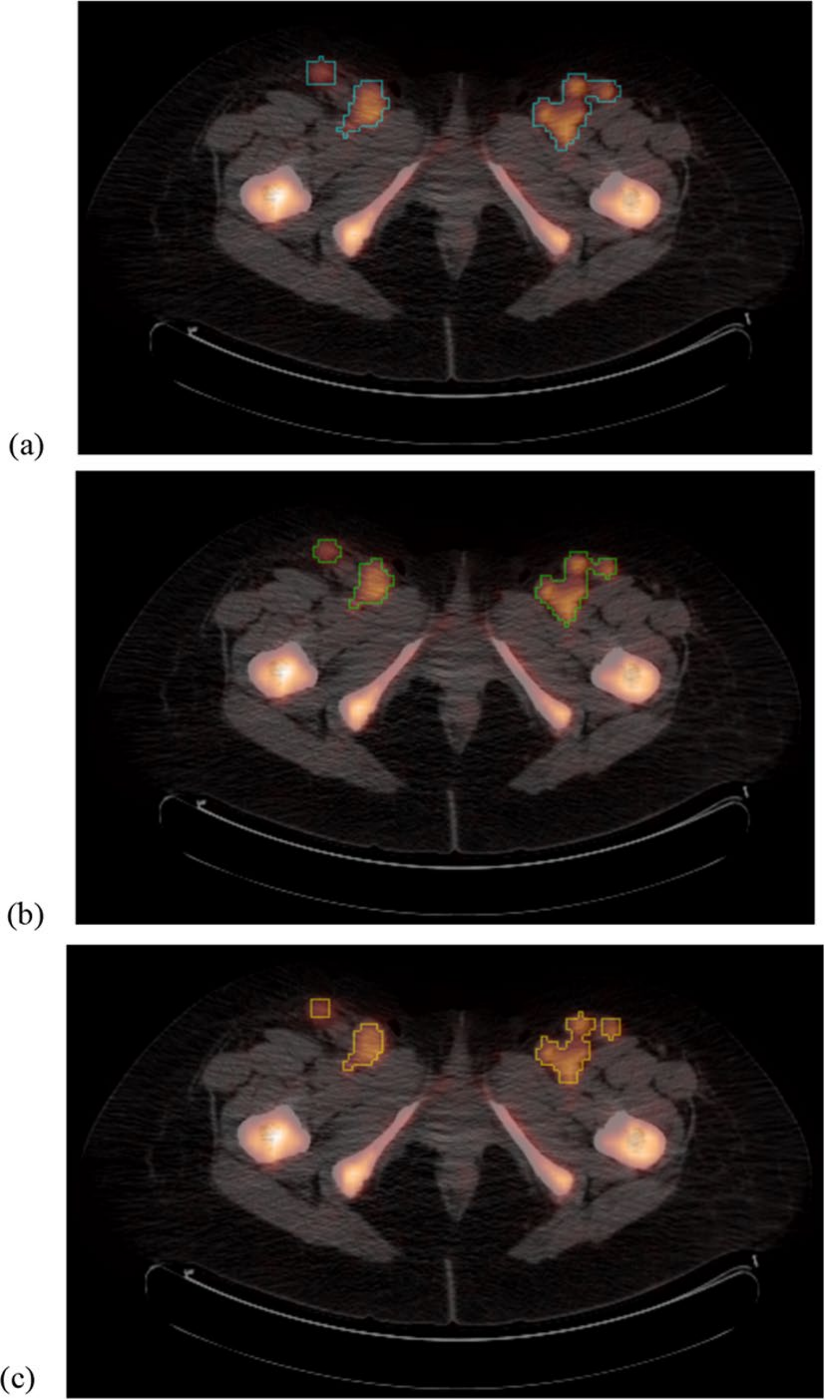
Example for comparing the limits of a lesion found by the three different criteria in a baseline PET/CT: a criterion 1; b criterion 2; and c criterion 3
